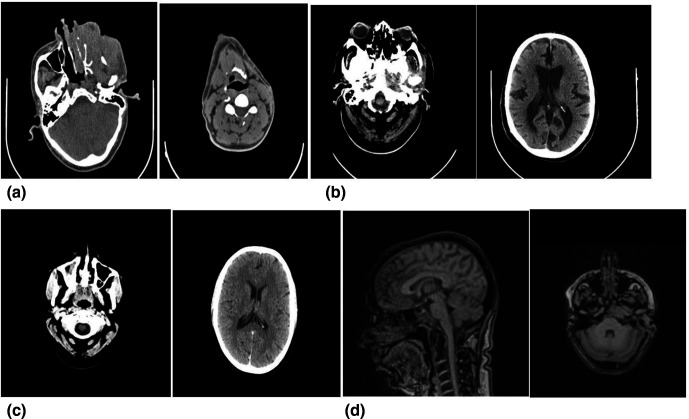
(a) A CT scan of case 1 shows infiltrative lesion of the left maxillary bone. (b) A CT scan of case 3 shows temporal bone soft tissue density – middle ear, mastoid with area of lysis and sclerosis on mandibular condyle. (c) A CT scan of case 5 shows temporal bone soft tissue density filling middle ear and mastoid. (d) A CT scan of case 7 shows post-operative cavity with destruction reaching petrous apex with enhancement along Meckel’s cave and cival region.
